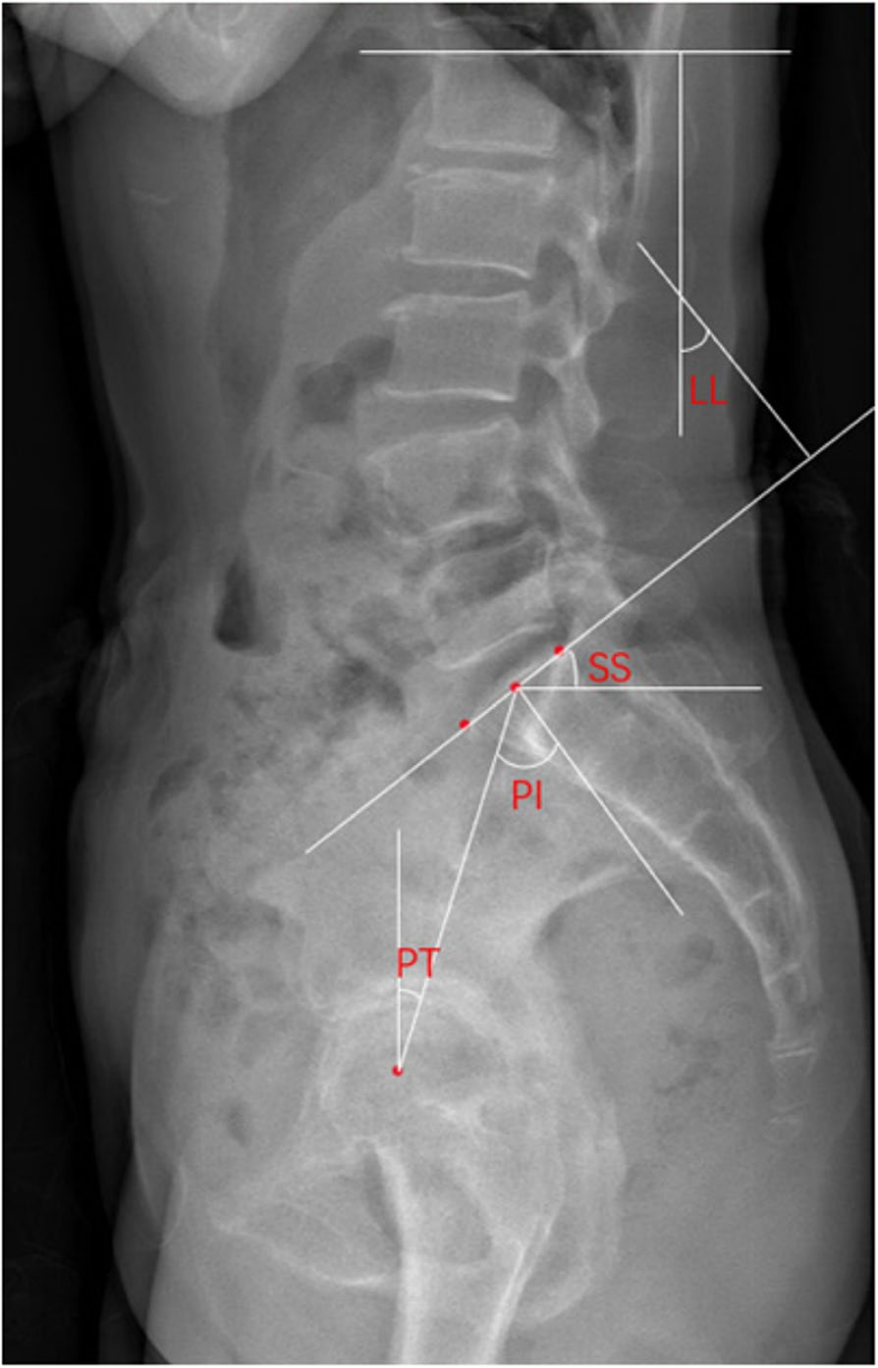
Plain lateral radiographs for measuring spino-pelvic sagittal parameters. LL: Lumbar lordosis, SS: Sacral slope, PI: Pelvic incidence, PT: Pelvic tilt
